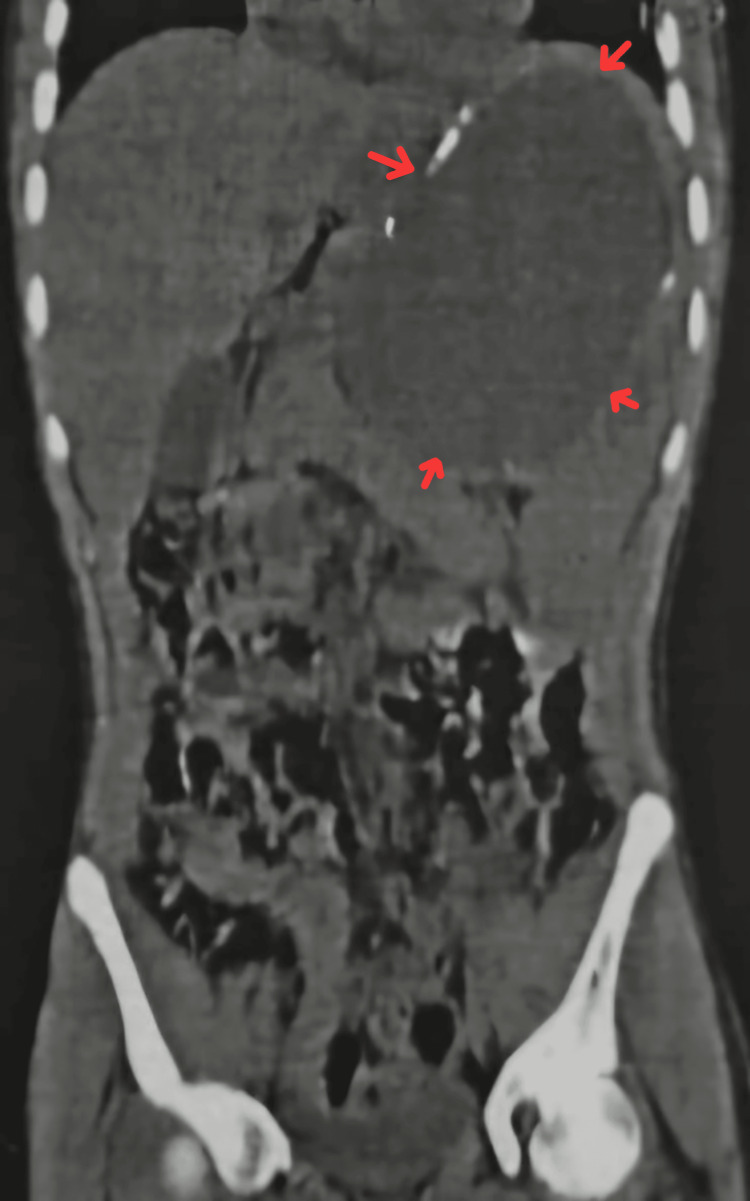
CECT of the abdomen (coronal view) This image shows gross splenomegaly with a large cystic lesion (red arrows), measuring approximately 1500 cc, with dense internal contents and a few lobulations within the splenic parenchyma at the upper and mid-pole, extending up to the subcapsular location. There is no solid component or enhancement. The cyst is seen abutting the inferior surface of the left lobe of the liver and the tail of the pancreas, with the stomach displaced to the right side and no encasement of the splenic vessels. CECT, Contrast-Enhanced Computed Tomography
